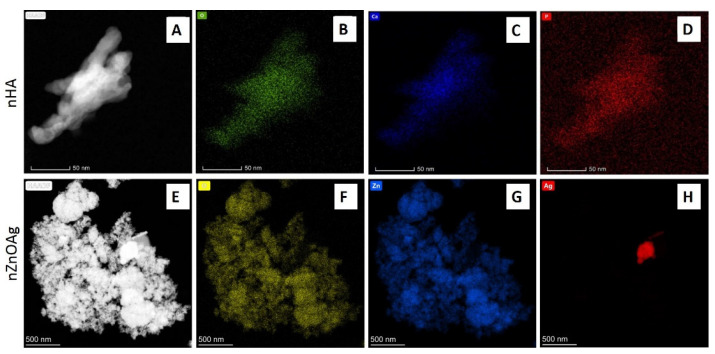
TEM images with energy-dispersive X-ray (EDX) mapping of different elements for nHA (A–D) and nZnO:Ag powders (E–H). For nHA TEM (top row), colors and complementary chemical elements on the map are as follows: (O—oxygen) green, (Ca—calcium) blue, (P—phosphorus) red. Respectively, for nZnO:Ag (bottom row), colors are: (O—oxygen) yellow, (Zn—zinc) blue and (Ag—silver) red.
